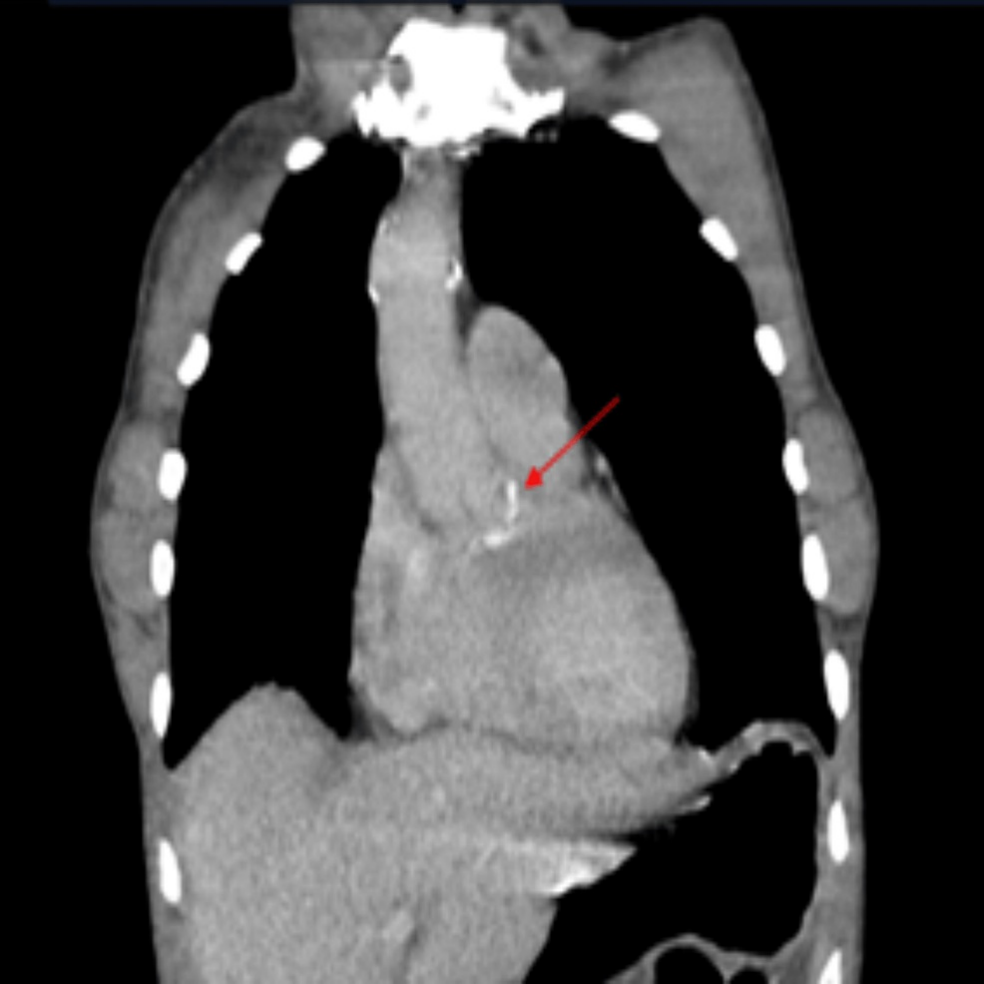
CT aortogram in the coronal plane, with red arrow highlighting site of aortic abscess.
